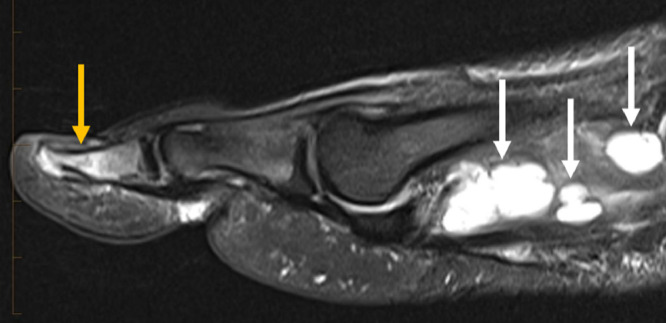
Sagittal T2 fat sat image from MRI of the right foot demonstrates edema-like marrow signal intensity in the hallux distal phalanx (orange arrow). Multiple fluid collections are noted in the forefoot (white arrows) (Color version of the figure is available online.)
